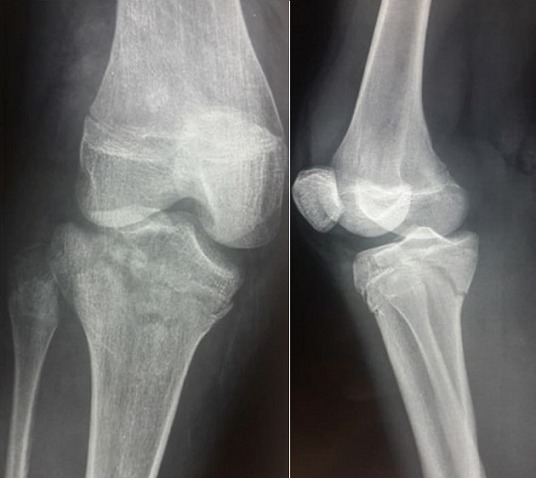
Radiographies standards de face et profil du genou droit révélant une fracture complexe de l’extrémité proximale du tibia
